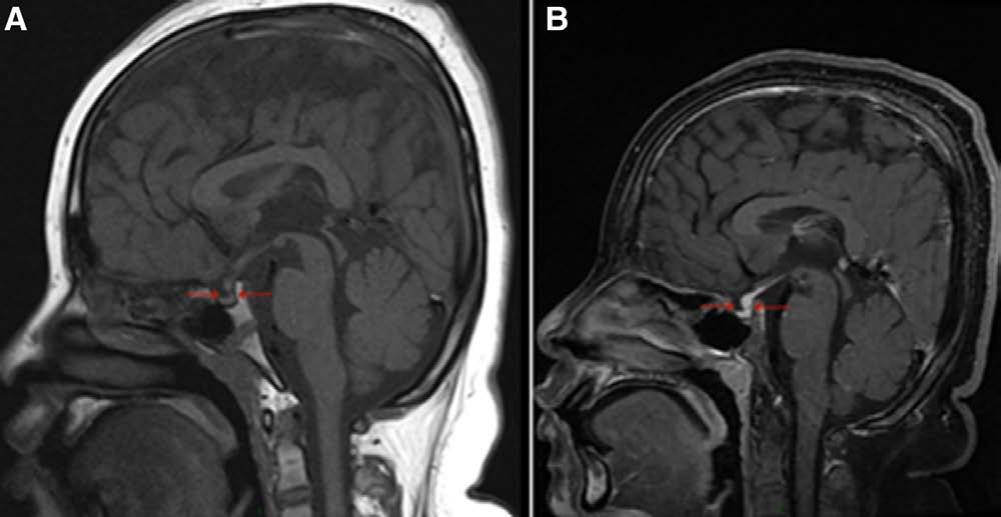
Pituitary hypoplasia (anterior pituitary dysplasia): (A) MRI scan of the pituitary gland: the pituitary stalk exhibits an enlarged diameter of approximately 4.2 mm, and there is an absence of a high signal in the neuropituitary gland on T1WI. (B) MRI-enhanced scan: the pituitary stalk appears slender in the lower part and thickened in the upper part, forming a nodular structure with a diameter of approximately 4.2 mm. MRI = magnetic resonance imaging.
